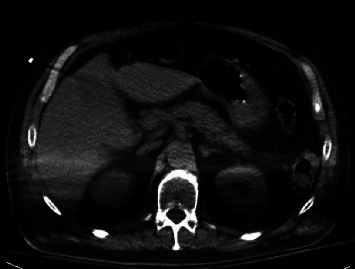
CT scan of the abdomen without evidence of gastric wall thickening or gas in the intramural layer.
